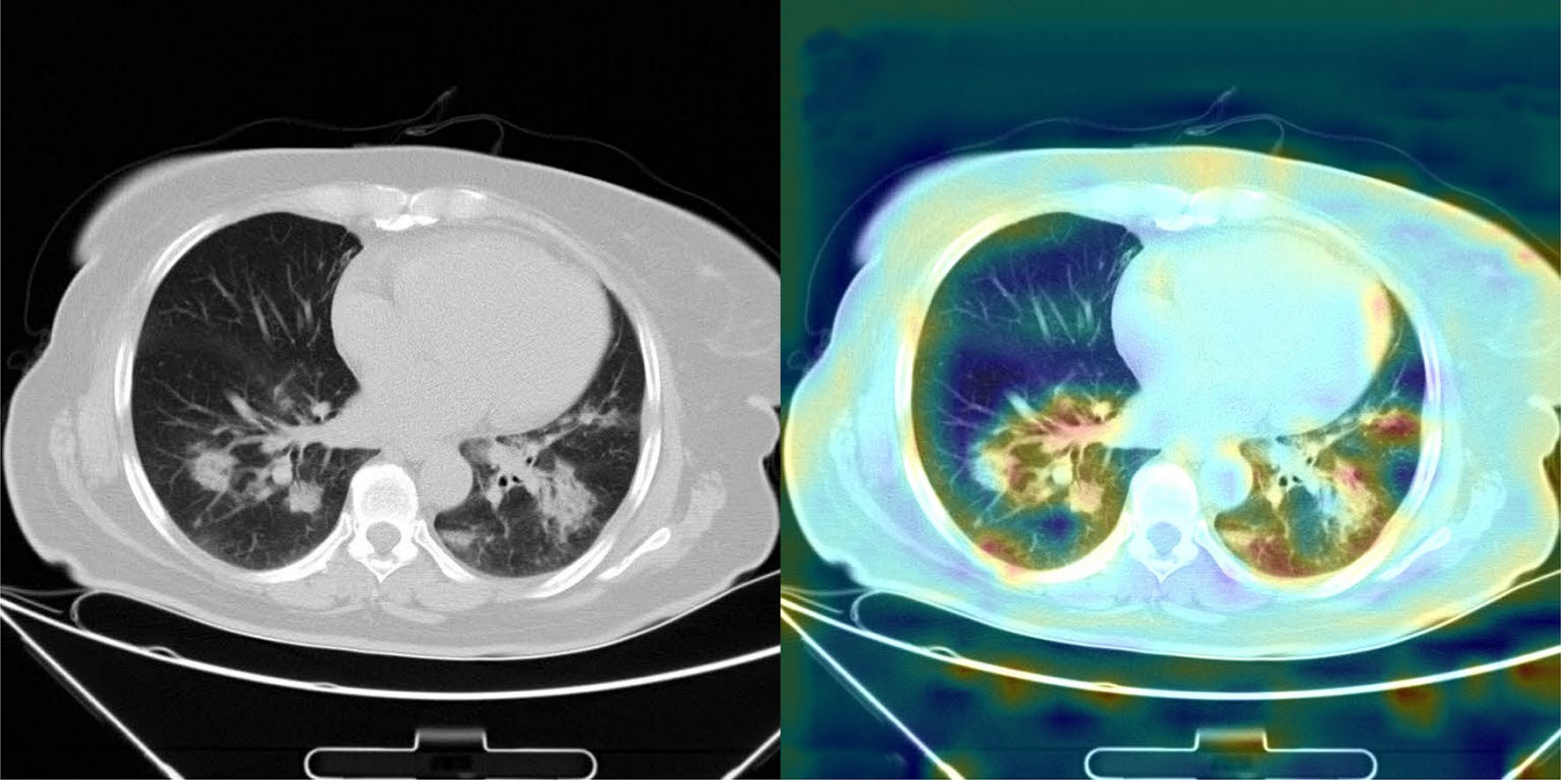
Plotting the results of Class Activation Mapping (CAM) for a CT-image instance of COVID-19 (left) based on Deep-CT-Net: The CAM image (right) highlights the class-specific discriminative/attention regions. The highlighted areas inside of the lungs are the discriminative regions for screening COVID-19.
